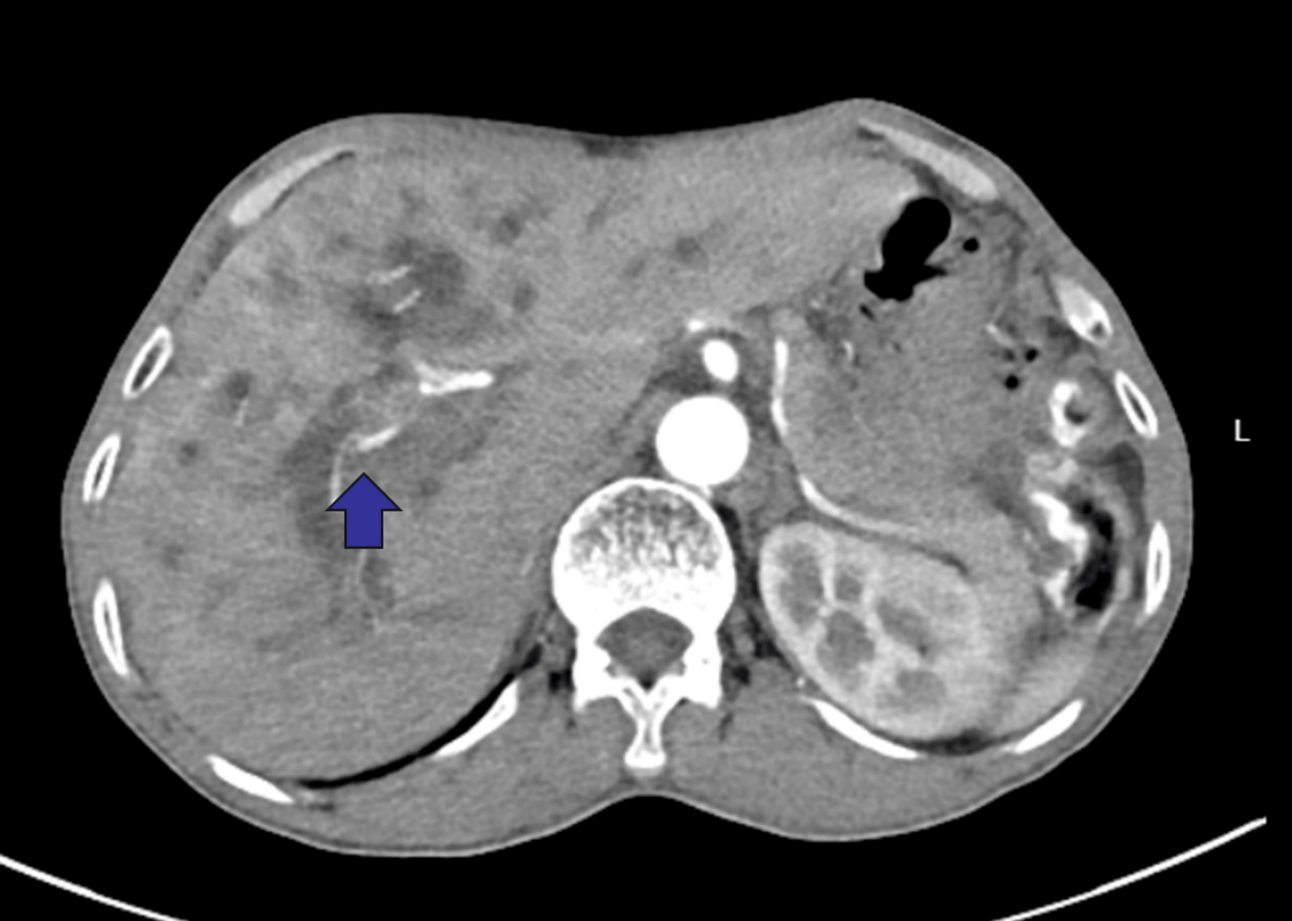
Contrast-enhanced CT showed dilation of intrahepatic and extrahepatic bile ducts (blue arrow)
